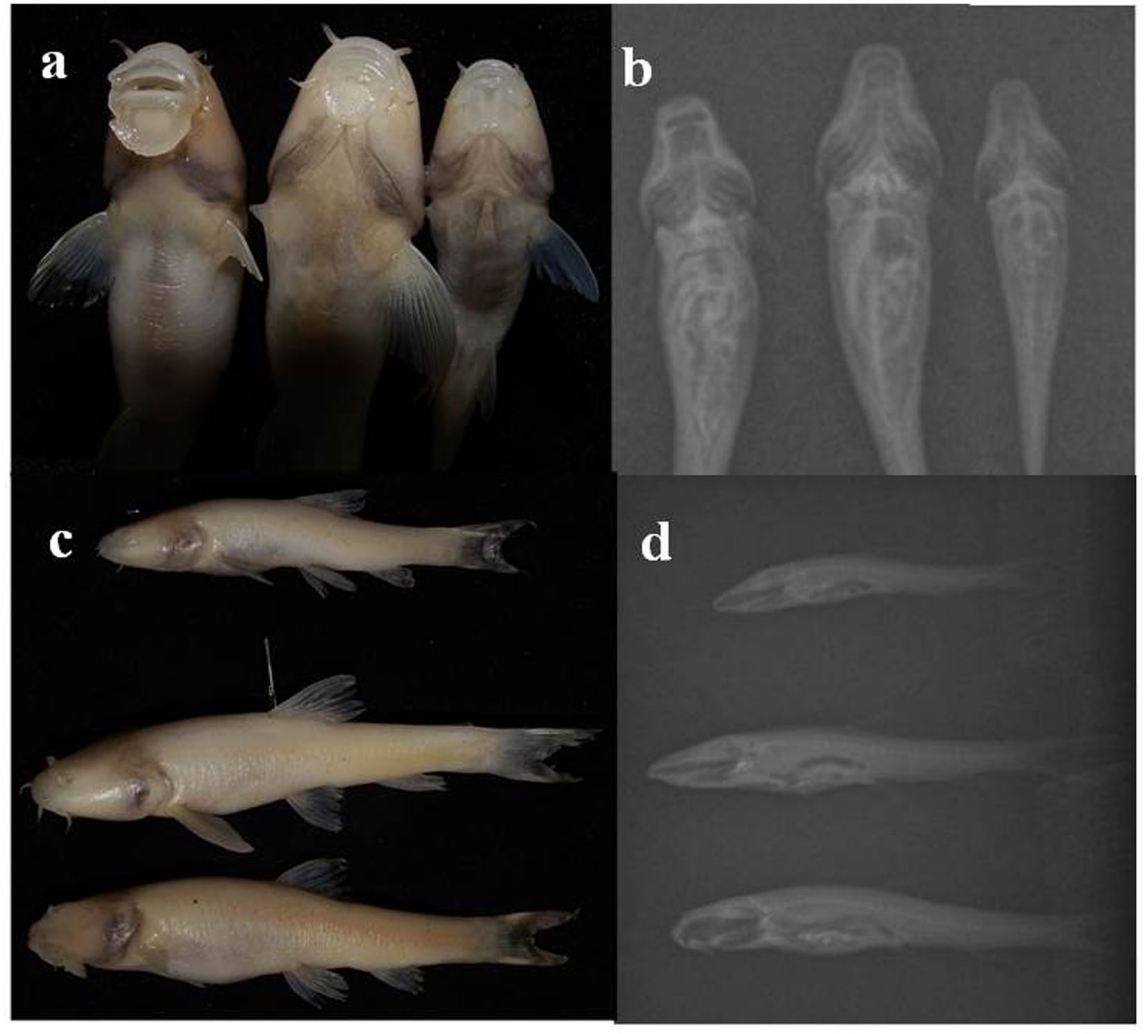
The different sympatric mental disc forms in blind Iran cave barb; (a): from right to left: well-developed disc, intermediate disc, and reduced disc, and (b): the corresponding ventral x-ray view of different disc forms; (c): from up to bottom: reduced disc, intermediate disc, and fully developed disc, and (d): the corresponding x-ray side view of different disc forms.
